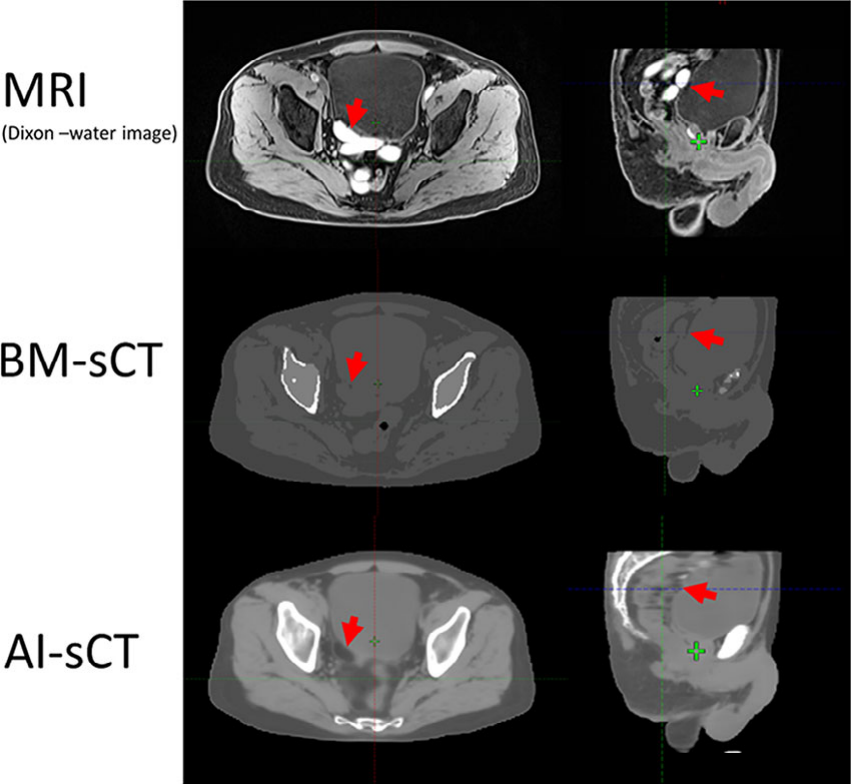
Example case of MRI sequence artifact causing missing bowel loops in AI‐sCT. Red arrows indicate corresponding bowel loops which show also a high‐intensity signal in the MRI Dixon‐water image, as soft tissue indistinguishable from the bladder wall in BM‐sCT and as visceral fat in AI‐sCT. AI, artificial intelligence; BM, bulk‐density‐method; CT, computed tomography; MRI, magnetic resonance; sCT, synthetic computed tomography.
